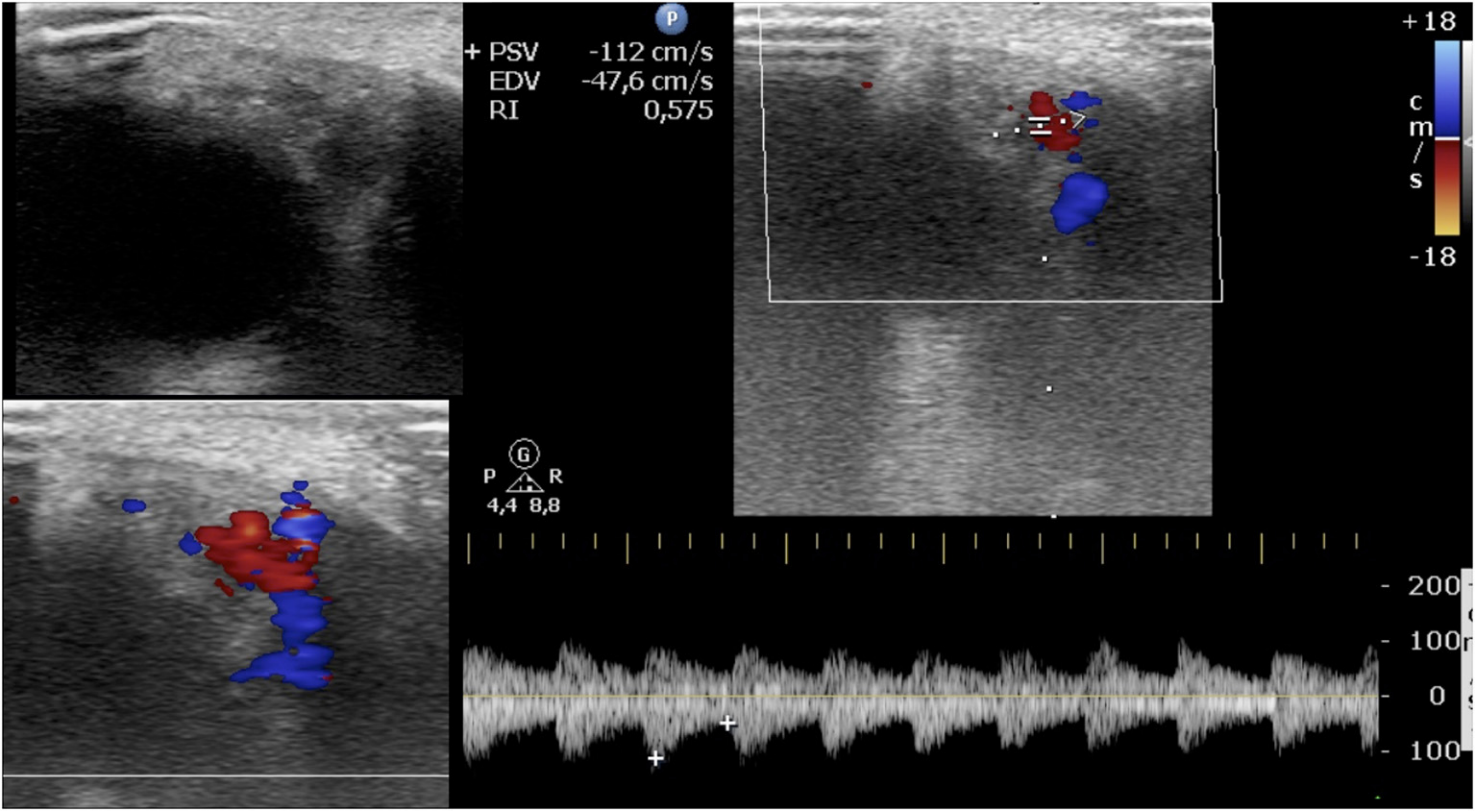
The same infantile hemangioma of Figure 1. Latest ultrasound evaluation with detectable color Doppler signals after 3 months of oral propranolol. Post-treatment images show a smaller mass with heterogeneous echogenicity; at color Doppler, the vascularization is reduced, and the resistive index is 0.57.
